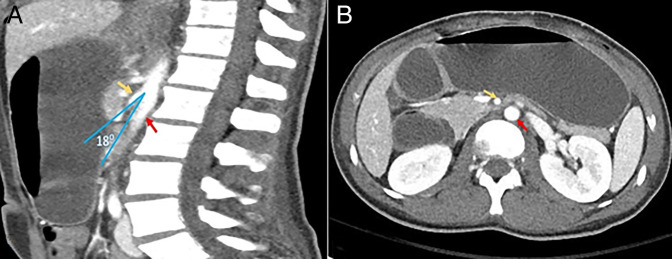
(A) Abdominal computed tomography sagittal view: Aortomesenteric angle = 18°; (B) dilatation of the stomach and the first part and second part of the duodenum, which transitions into decompressed bowel loops beyond the SMA. Yellow arrow = SMA; red arrow = aorta. SMA, superior mesenteric artery.
